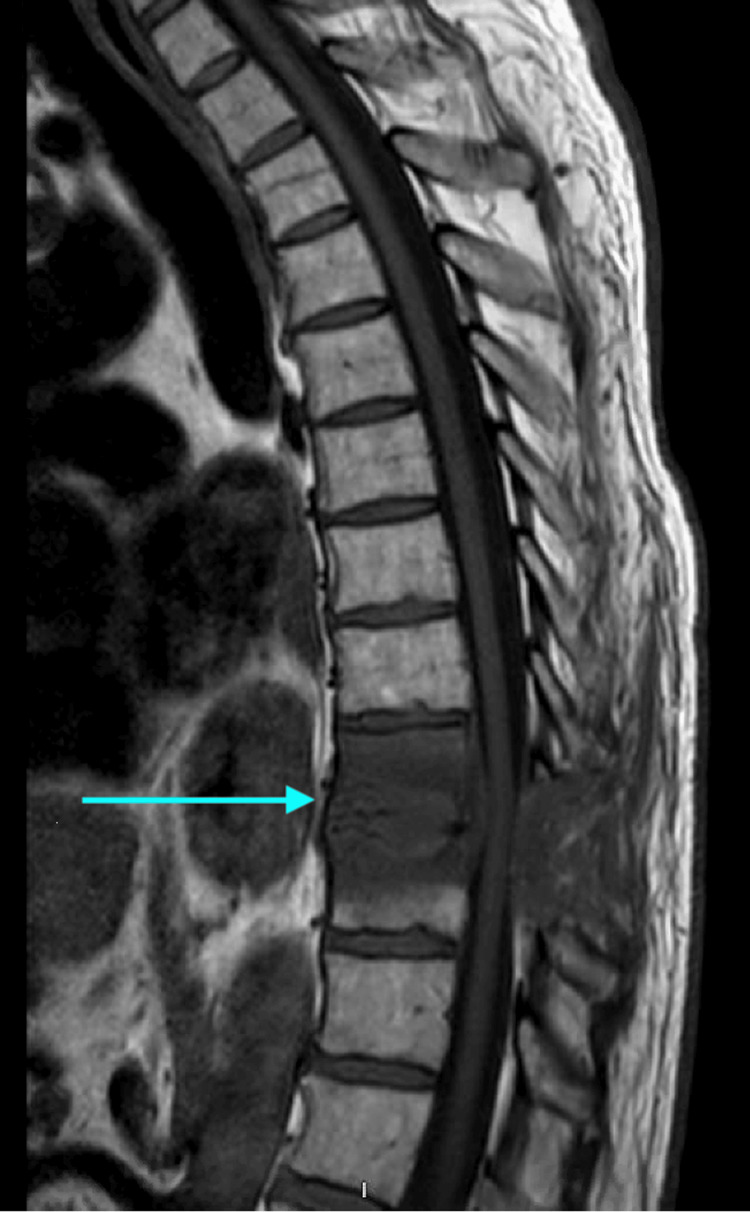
MRI of the thoracic spine with the T9-T10 area being involved.
